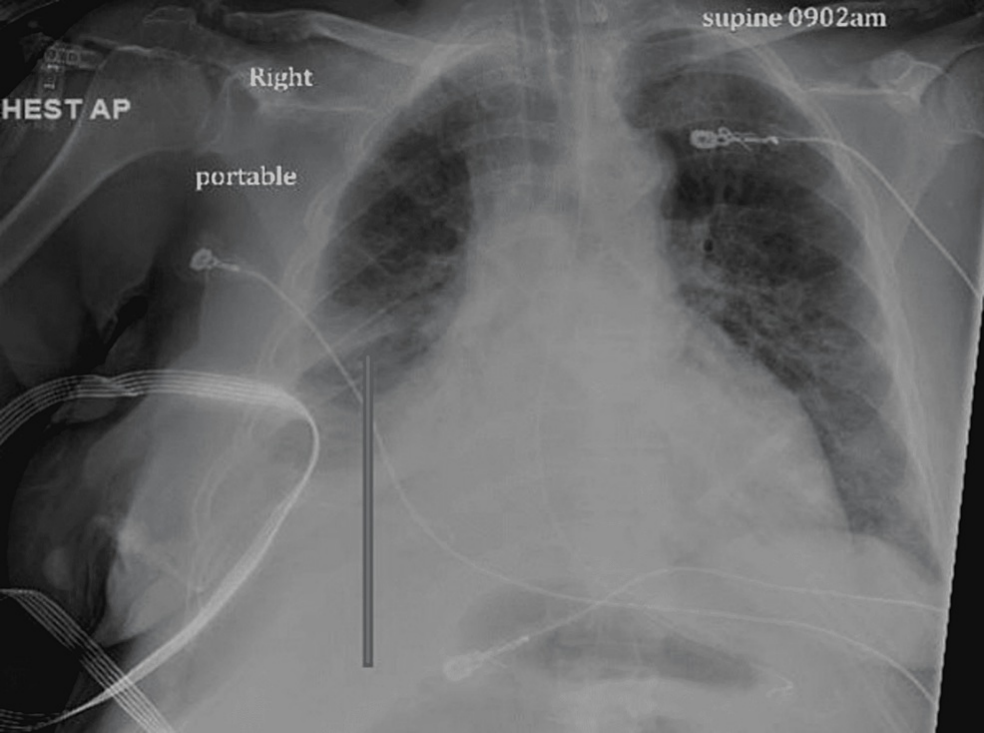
Portable Chest X-ray anteroposterior view taken after three days of chest tube drainage showing significant improvement compared to previous imaging (arrow).
